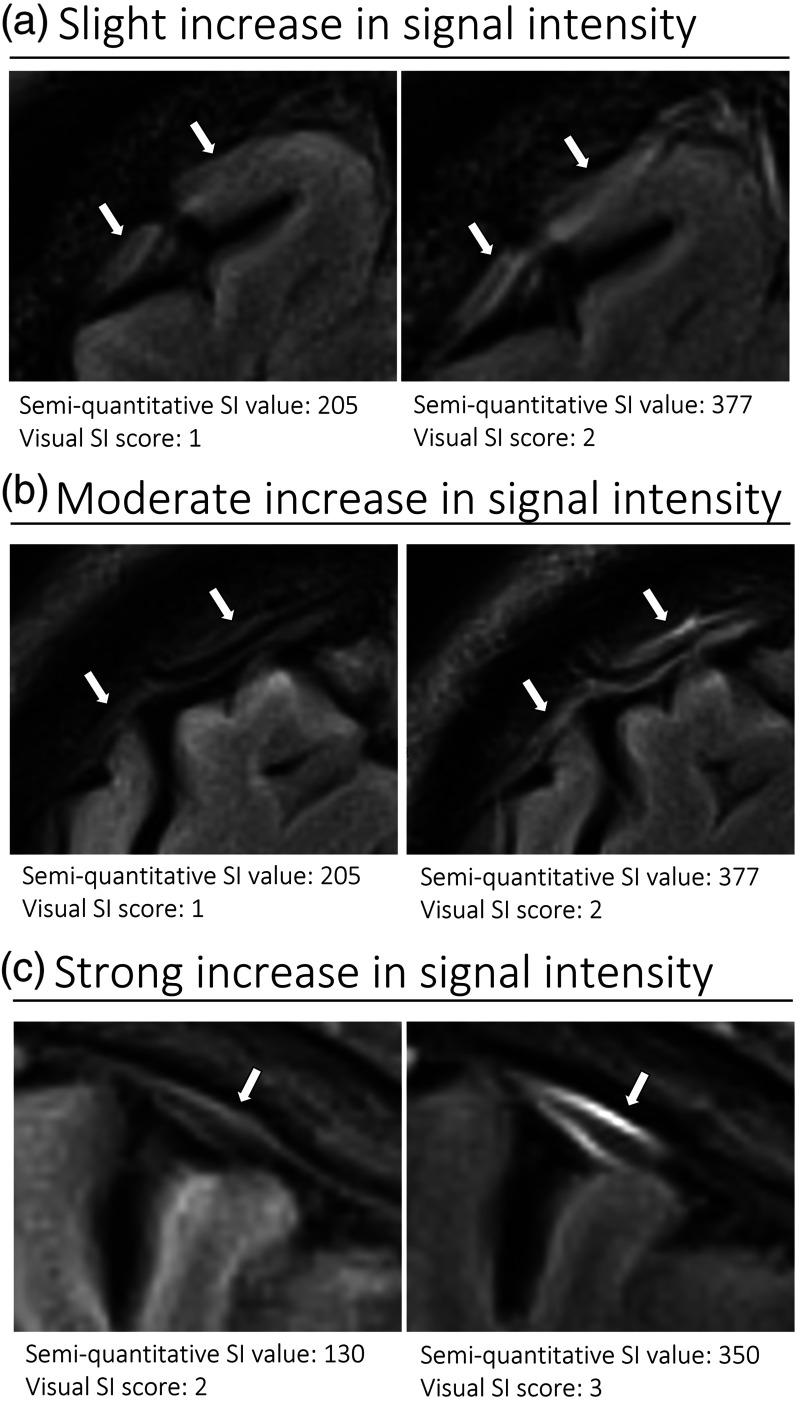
Representative T2 FLAIR images of superficial cortical perivenous spaces. Pre- (left side of all panels) and post-contrast (right side of all panels) images in the same subject showing slight (a), moderate (b), and strong (c) increase in SI (different subjects for a, b, and c). The measured semi-quantitative SI values in arbitrary units (the average of all regions of interest measured at the specific area) and qualitative, visual SI scores are indicated below the images. The arrows indicate the perivenous spaces. SI: signal intensity.
